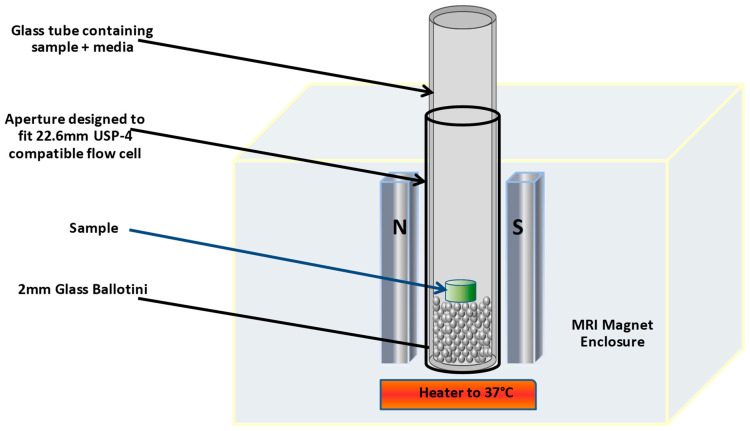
MARAN-i low-field magnetic resonance imager, with static cell set-up.
